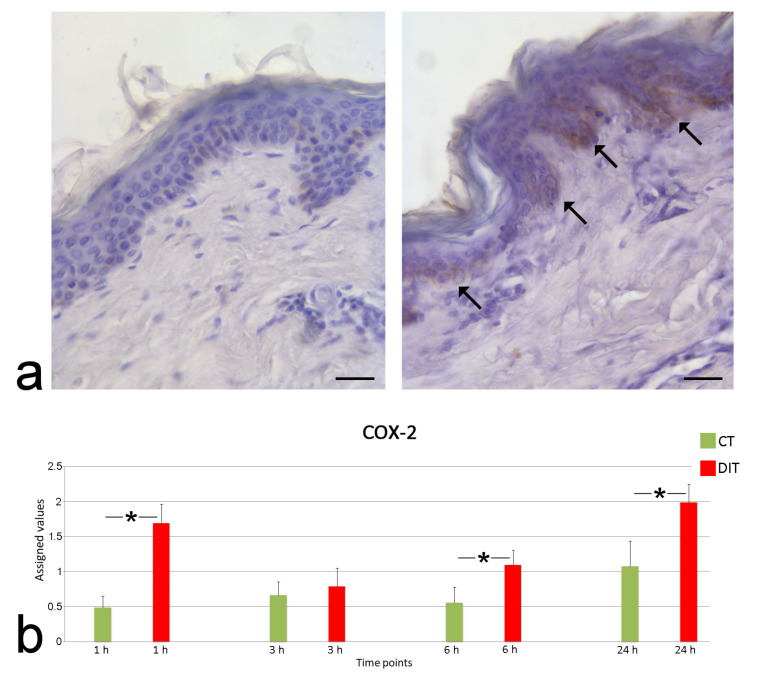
(a) Skin sample untreated (left) and treated with dithranol for 1 h (right); immunolabelling with anti-COX-2 antibody. Keratinocytes of the treated sample show an evident signal (arrows). Bars: 50 µm. (b) Mean ± standard error values of labeling values. Asterisks indicate significant difference (3 h CT vs. 3 h DIT, *p* < 0.001; 6 h CT vs. 6 h DIT, *p* = 0.013; 24 h CT vs. 24 h DIT, *p* = 0.009). CT, control (untreated); DIT, dithranol-treated.
